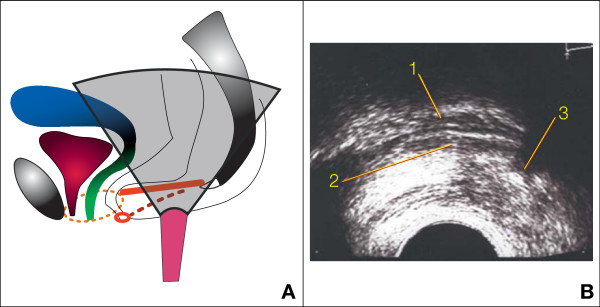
Retro-anal ultrasound of the levator plate. A: position of the probe between anus and coccyx (levator plate or ano-coccygeal raphe in red). B: ultrasonographic image: 1 = posterior rectal wall, 2 = levator plate, 3 = coccyx. Dotted lines represent structures which are not in the section plane: thin lines = limits of the levator hiatus, thick line = right pudendal nerve. Small red ring = anal sphincter.
